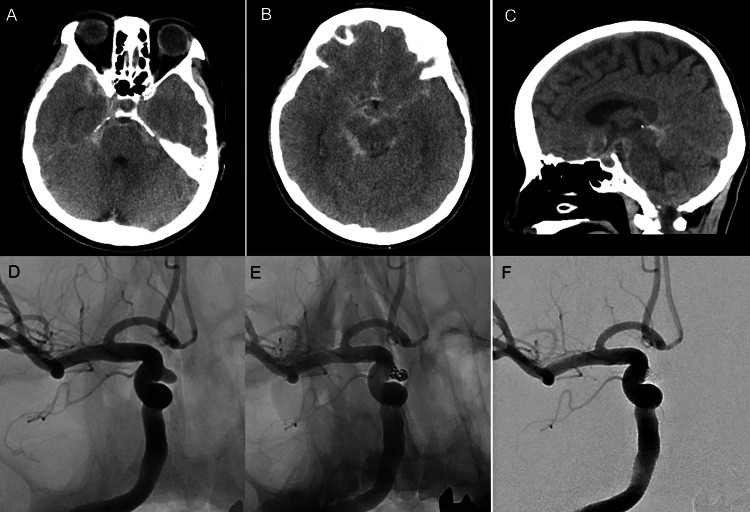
Initial head computed tomography (CT) and intra-aneurysmal coil embolization. (A-C) Head CT on admission. Subarachnoid hemorrhage extended from the prepontine and basal cisterns to the quadrigeminal cistern. (D) Right internal carotid artery (ICA) angiography on admission revealed a right internal carotid artery-superior hypophyseal artery (ICA-SHA) aneurysm. The ICA-SHA aneurysm was considered to have ruptured. (E, F) Subsequently, balloon-assisted coil embolization was performed, resulting in complete obliteration.
